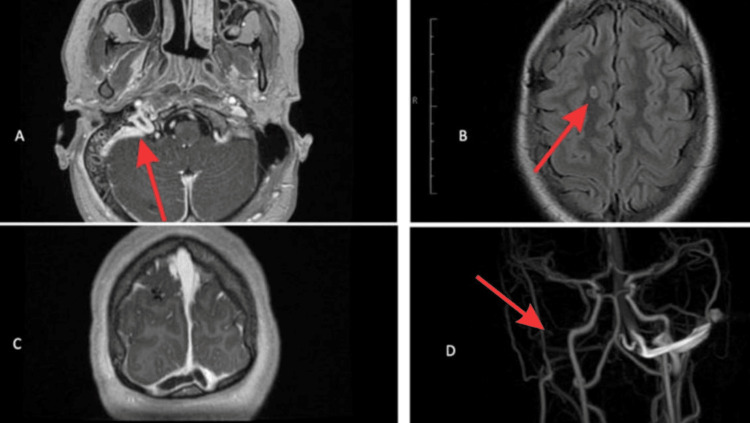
Contrast MRI findings. (A, C) indicate the presence of right ostomastoiditis complicated by thrombotic sequelae affecting the trochlea, as well as the proximal portion of the left transverse sinus (C), right transverse sinus, right sigmoid sinus, and the visible portion of the right internal jugular vein (A). The non-contrast FLAIR axial image (B) reveals the presence of an oval-shaped nodule with intermediate intensity in the right parietal subcortical/parasagittal white matter. Additionally, the MRV  (D) shows a lack of visualization of the right transverse and sigmoid sinus, as well as the internal jugular vein FLAIR: fluid-attenuated inversion recovery; MRV: magnetic resonance venography
